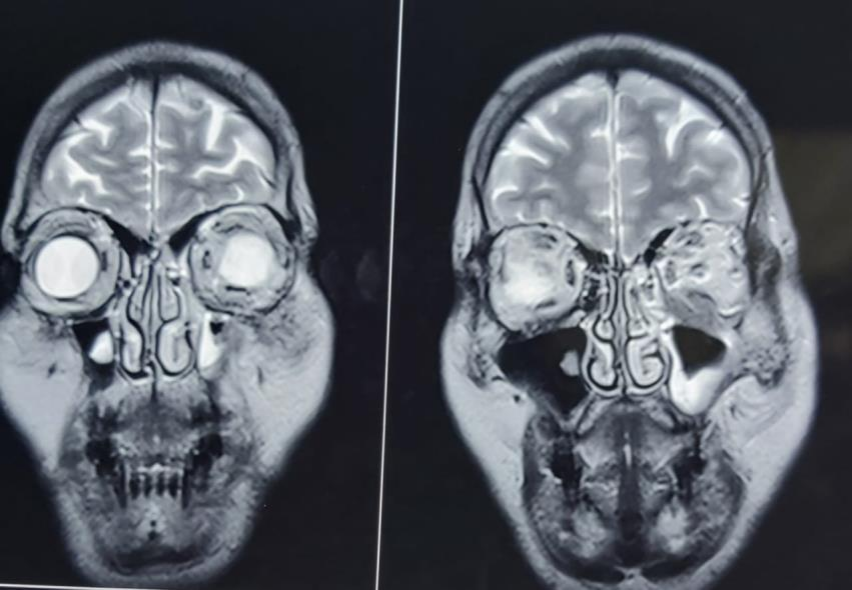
Coronal section of MRI PNS shows moderate mucosal thickening of the maxillary sinus along with soft tissue in left sided middle ethmoidal air cells, eroding the medial orbital wall. Hypertrophied middle and inferior turbinates can also be seen
